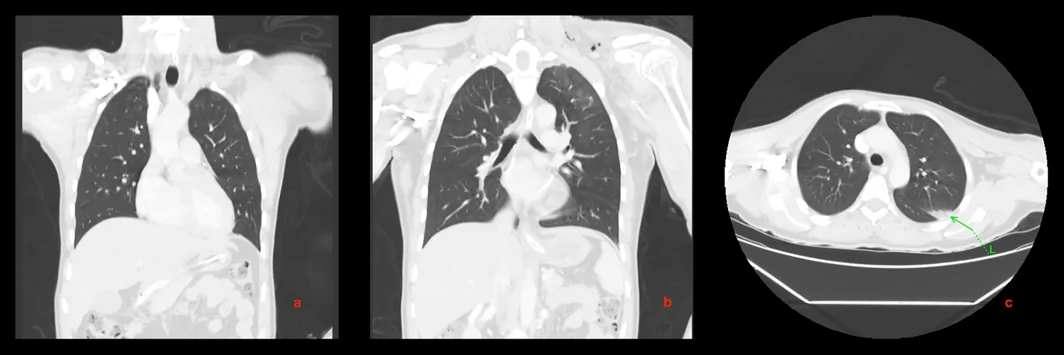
Sagittal & Axial chest CT image in lung window demonstrates nodular and coalescent ground‐glass opacities (GGOs) in the left upper lobe, consistent with pulmonary contusion and hemorrhage (arrow in c). Note is also made of left superficial neck subcutaneous emphysema.
